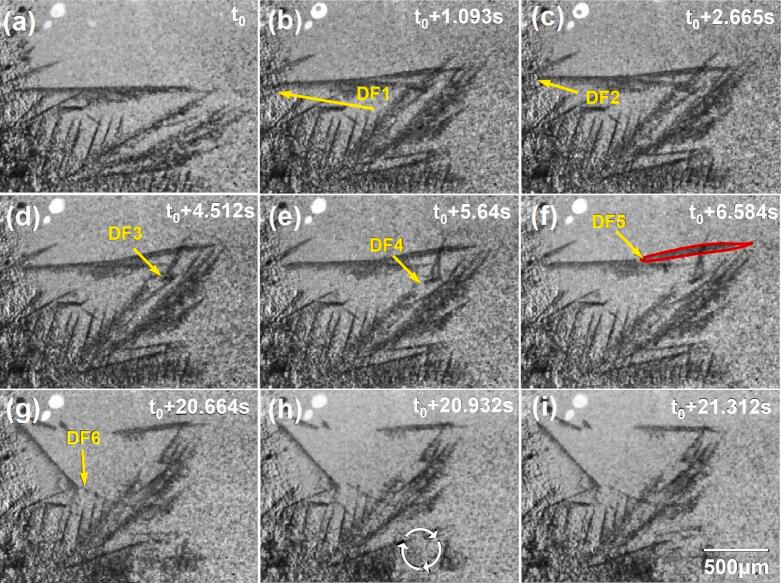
(a) − (f) dendrite arm fragmentation due to the cyclic fatigue effect induced by the acoustic flow. (g) − (i) dendritic fragmentation caused by the swirling flow. Experimental conditions: 30 W ultrasound, 1137.5 K/m thermal gradient, 250 fps (see Video 3).
